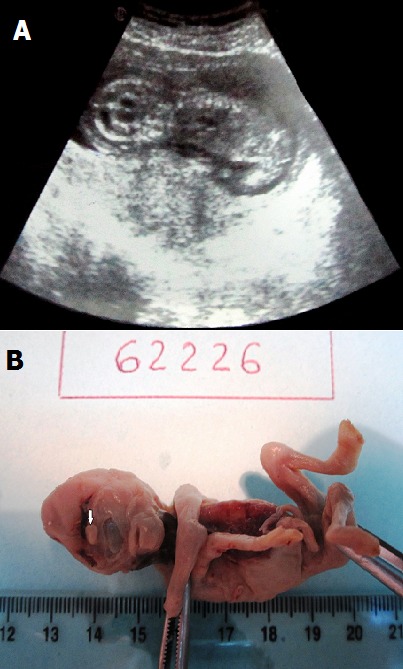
(A) ultrasonography revealed a retention image measuring 67,2 mm with no cardiac pulsations; (B) the fetus was monophtalmic with completely fused eyes. In the face, there was a central proboscis (white arrow) located above the single orbital cavity. The nasal structures were absenT
